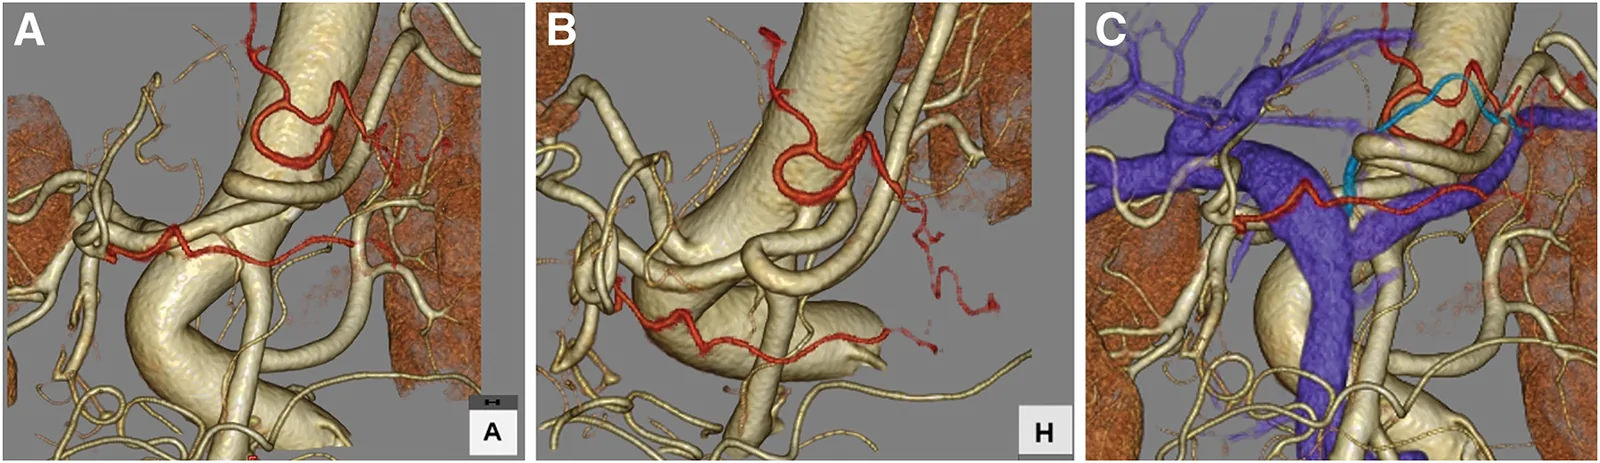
Preoperative 3D-CTA. (A, B) The left gastric, splenic, common hepatic, and superior mesenteric arteries arise independently from the abdominal aorta. (C) 3D reconstruction demonstrating the spatial relationship between the CHA and the portal vein, with the CHA coursing dorsal to the portal vein. CHA, common hepatic artery
